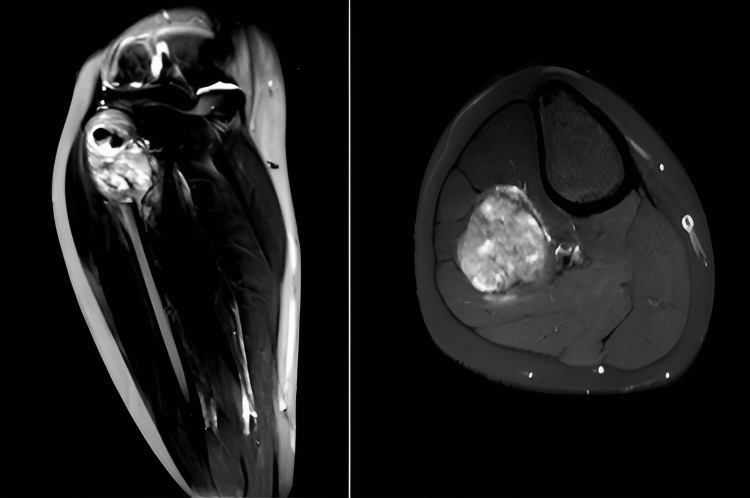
MRI showing a mass consistent with osteosarcoma localized in the proximal fibula and showing soft tissue expansion
